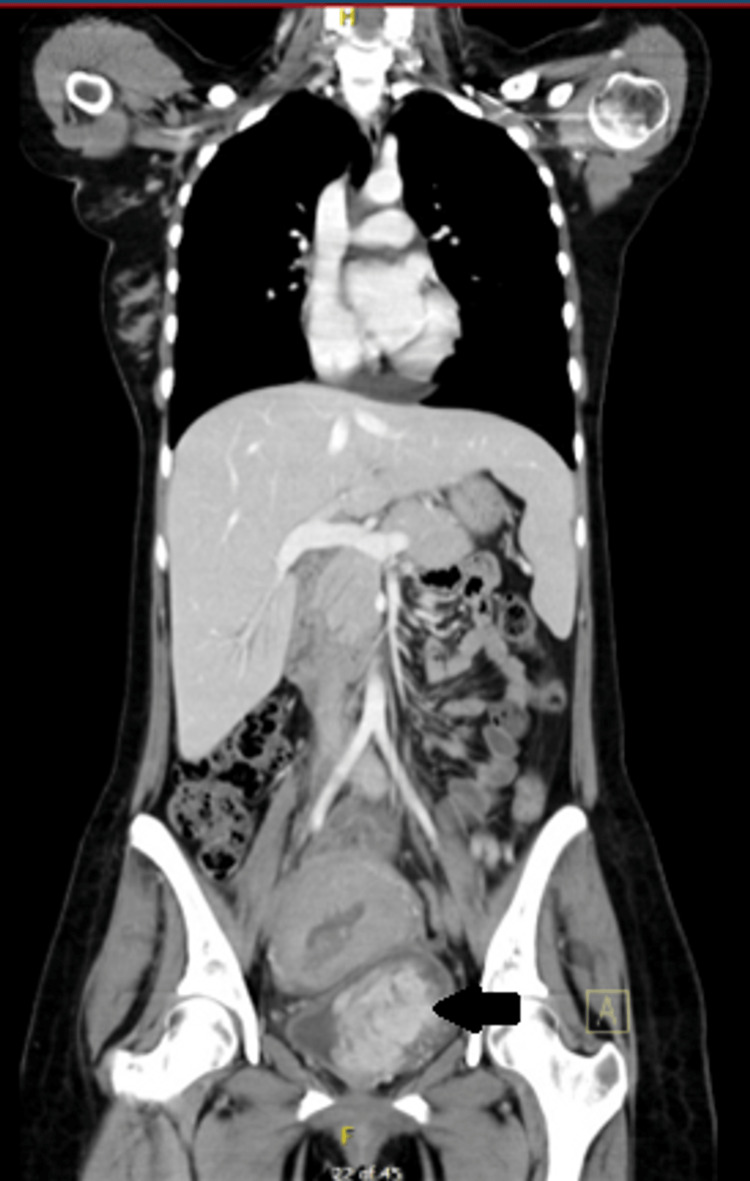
CT of the abdomen and pelvis (coronal section) showing a large irregular soft tissue mass arising from the left lateral wall and base of the bladder, measuring 6.1 x 6.0 x 6.0 cm, and encasing the right ureter at the ureterovesical junction. The uterus is anteverted and bulky, measuring 10.8 cm. CT, computed tomography
